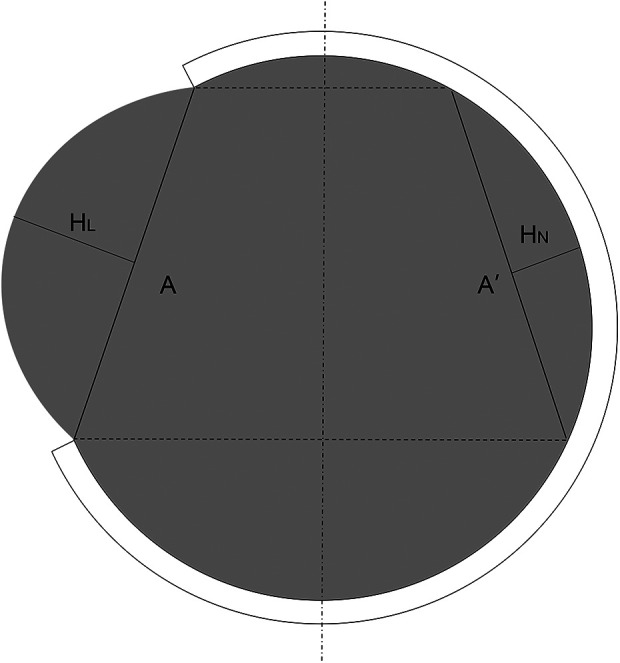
Measurement of herniation volume on maximal hernial layer of CT (A: diameter of craniectomy; H_L_: maximum perpendicular distance from hernial brain tissue to line A on lesion side. H_N_: maximum perpendicular distance from normal brain tissue to symmetrical line A’ on non-lesion sides).
